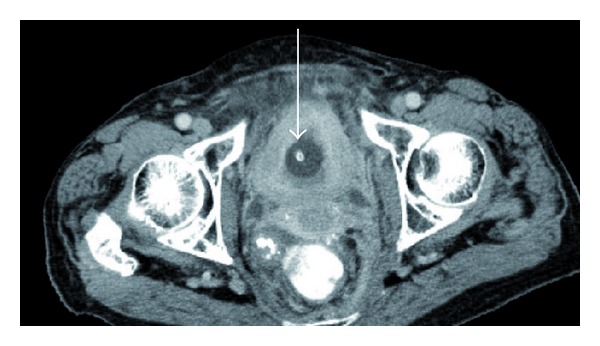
Axial computed tomography scan of the pelvis showing a diffuse infiltration of the bladder wall, catheter in situ (arrow).
